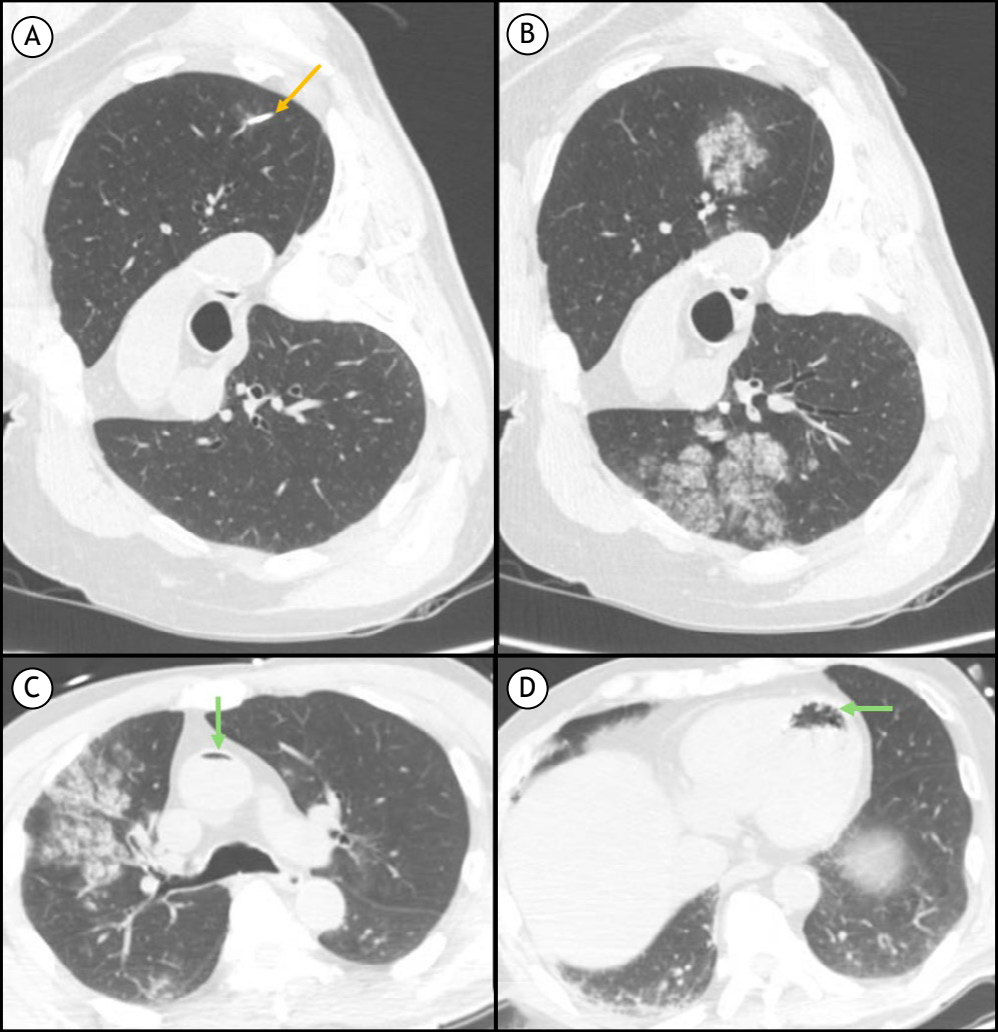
Axial CT slices. Slices A and B were acquired in the right lateral position. (A) The needle tip is within the lung nodule (orange arrow). (B) A few minutes after the needle biopsy, hemorrhage was observed surrounding the nodule in the left lung, as well as in the right lung, presumably due to contralateral aspiration favored by the right lateral position. Slices C and D were acquired in the supine position, at 15 m after the biopsy, and show a small amount of air in the ascending aorta, as well as air within the left ventricle (green arrows).
